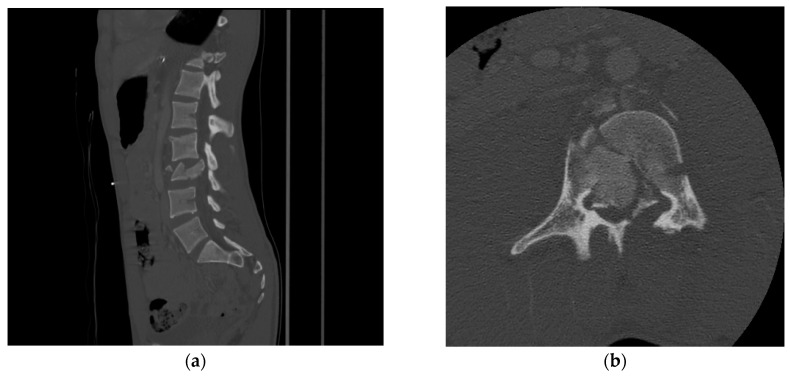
(a) Sagittal and (b) axial view of CT showing L3 burst fracture with significant retropulsion of the vertebral body into the spinal canal and loss of body heigh. There is involvement of the posterior elements.
